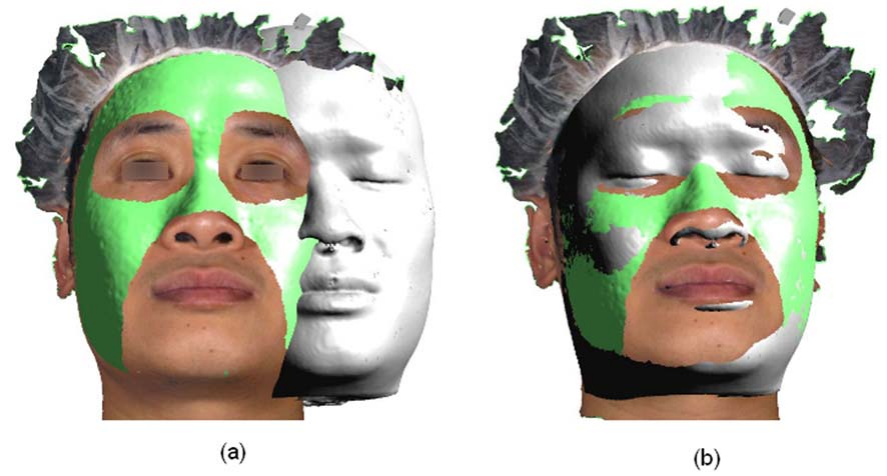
The matching of Cone-beam CT soft tissue and stereophotograhic images during registration. Initial manual aligning (a) is followed by automated registration (b).
